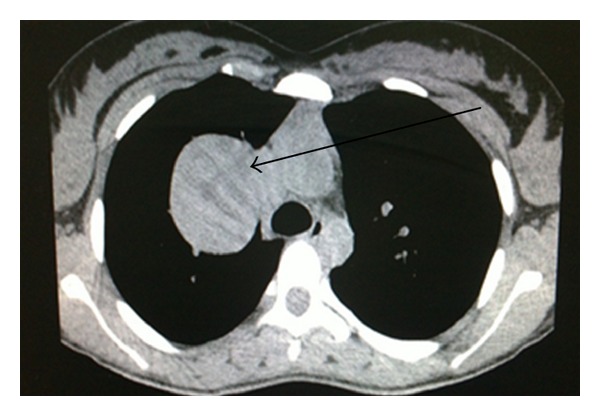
CT scan showing hypodensity (arrow) at the right upper lobe with significant compression to the superior venacava.
